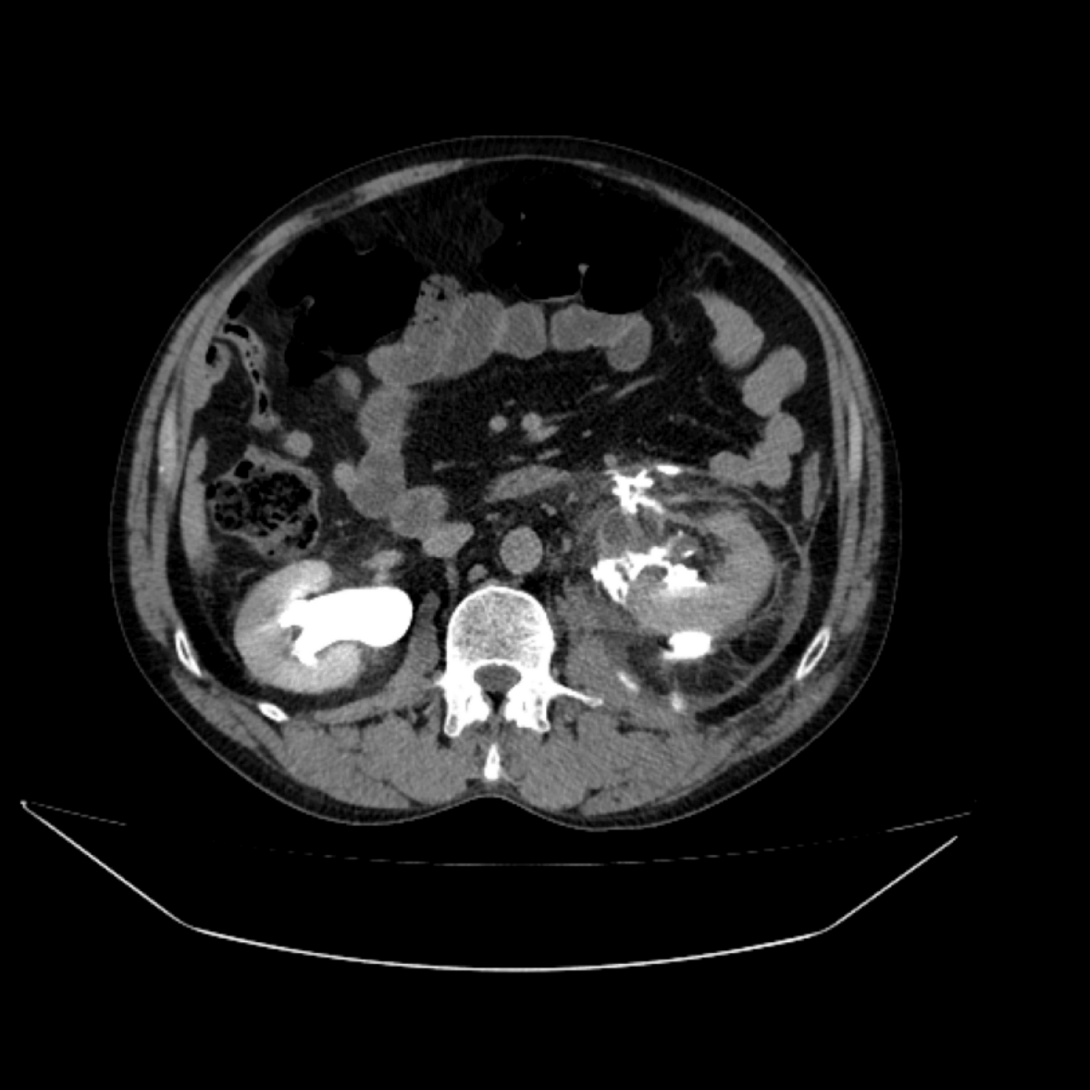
Contrast-enhanced CT image showing the presence of urinary extravasation at the level of the left upper lumbar ureter
